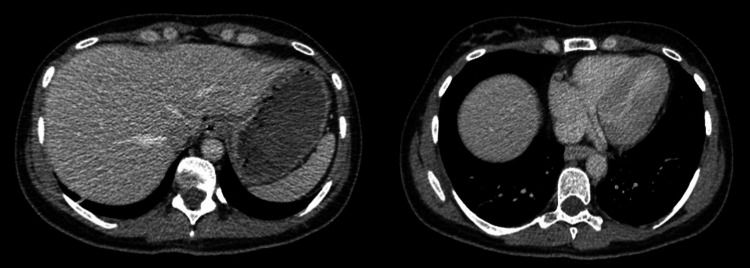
Follow-up CT showing complete resolution of HPVG CT: computed tomography; HPVG: hepatic portal venous gas
